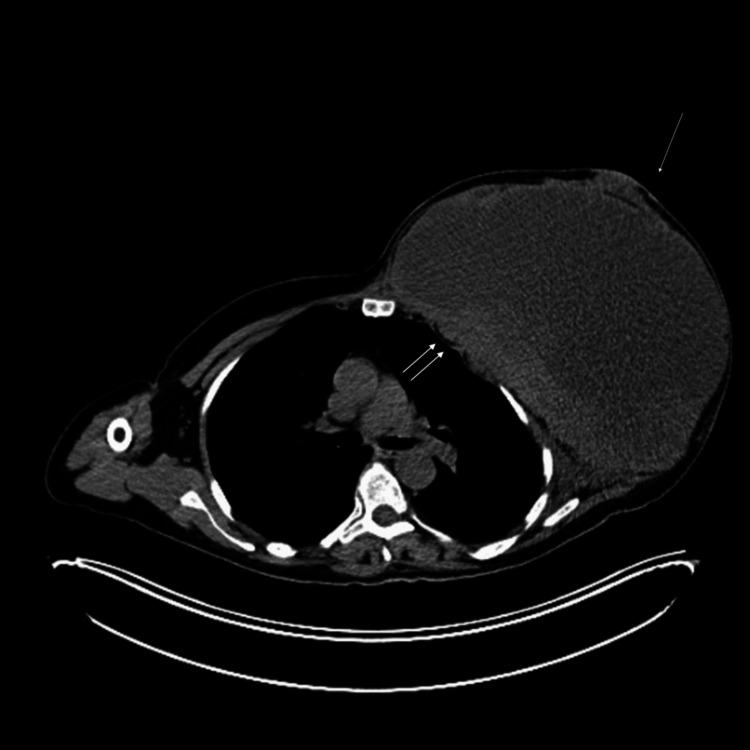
The PET-CT revealing a huge left breast mass. The single arrow points at the lesion, and the double arrows point at the attachment and invasion to the chest wall.
